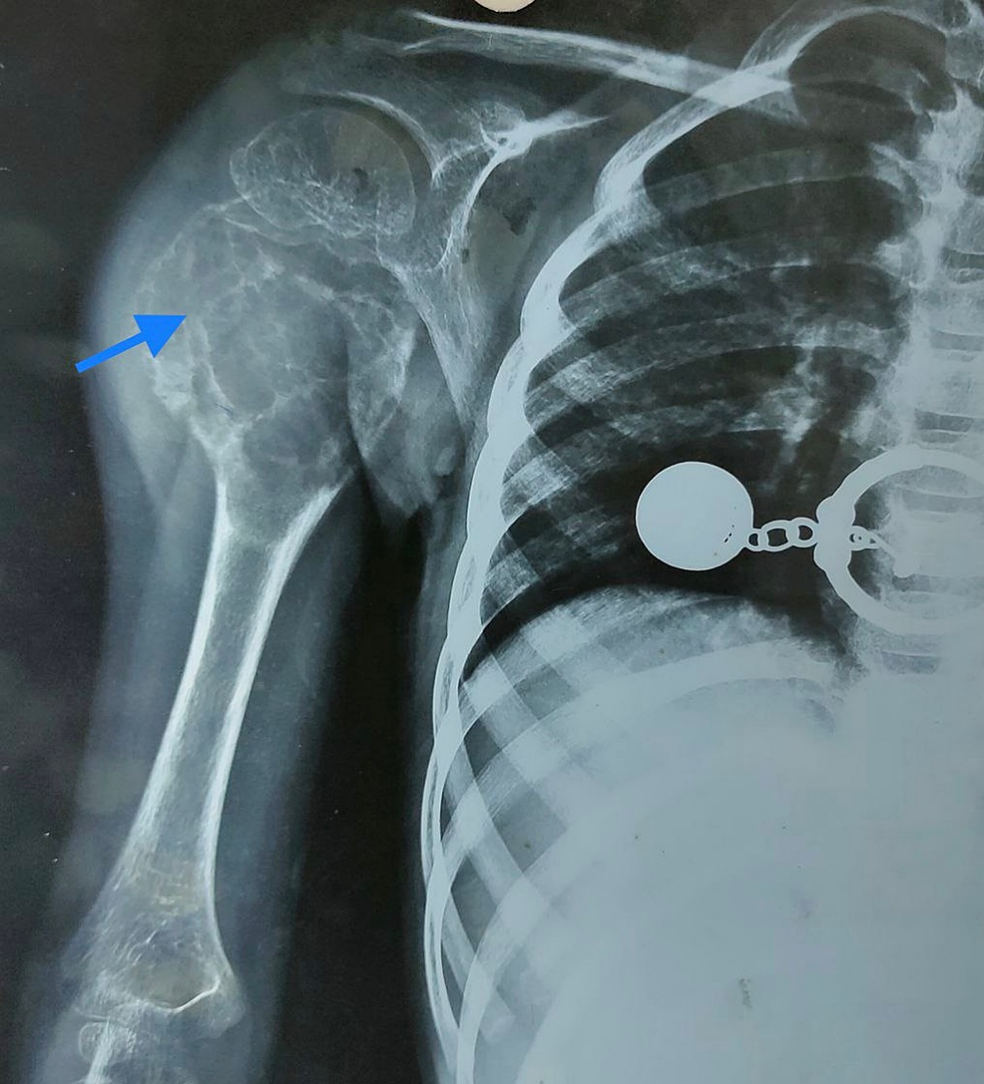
Plain radiograph (anteroposterior view) showimg a lytic lesion in the proximal portion of the right humerus.
